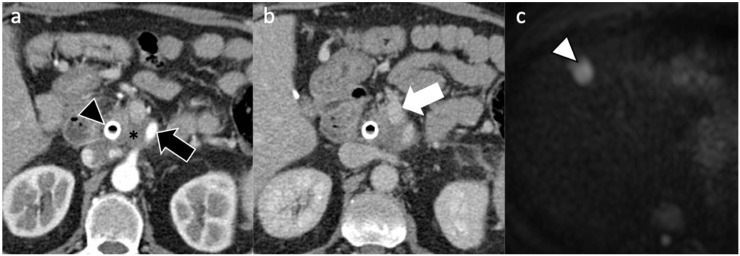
A 61-year-old man with non-resectable PDAC. Pancreatic CT scan on (a) arterial and (b) portal venous phases shows the presence of a biliary stent (black arrowhead) and a pancreatic mass (*) causing encasement of both superior mesenteric artery (black arrow) and vein (white arrow). The patient commenced modified FOLFIRINOX regimen at diagnosis. However, after 6 months, (c) liver MRI on diffusion weighted imaging showed appearance of liver metastasis in segment IV (arrowhead in c).
